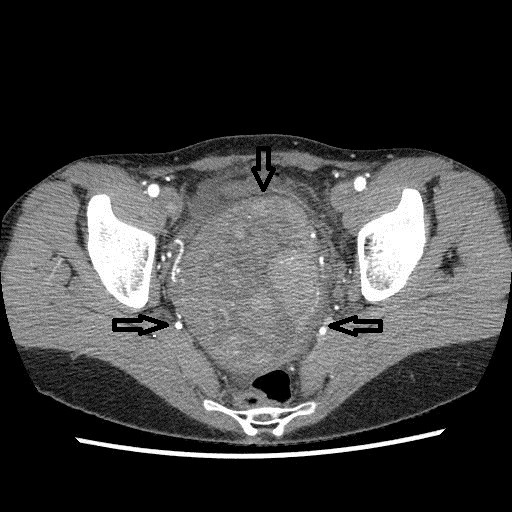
Axial computed tomography scan of the pelvis. A large mass arising from the cervix (arrows) and compressing the bladder and the rectum.
